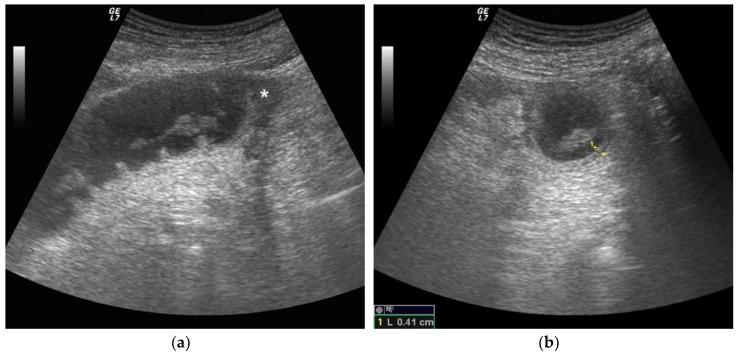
A 68-year-old female patient with previous abdominal surgery. Ultrasound image long (a) and axial (b) evaluation of a fluid-filled dilated small bowel loop with hyperechogenic floating material. Bowel peristalsis was absent. Mild parietal and valvulae conniventes thickening were present. Free fluid between bowel loops was detected (*). At surgery, mechanical obstruction due to a bridle was evident after release of adhesion, the bowel loop pinks up, peristalsis recovered and resection was avoided.
